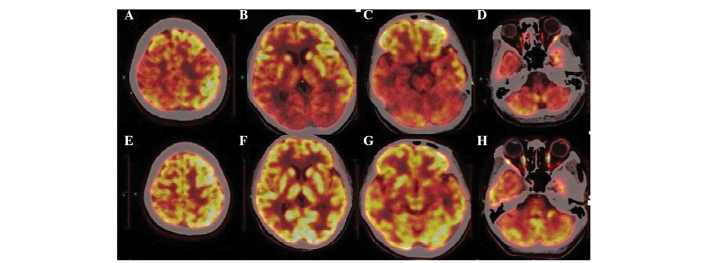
Selected images of the ^18^F-FDG PET brain scans. (A–D) Prior to treatment with high-dose corticosteroids and plasmapheresis, the scans revealed generalized hypometabolism without significant hypermetabolic foci. A significant asymmetrical reduction in the metabolism between the left and right hemispheres was noted, particularly in the temporal and parietal cortex. (E–H) The metabolic activities of the former areas were normalized following treatment, with the exception of a slightly lower metabolism in the right temporal cortex when compared with the contralateral site.
